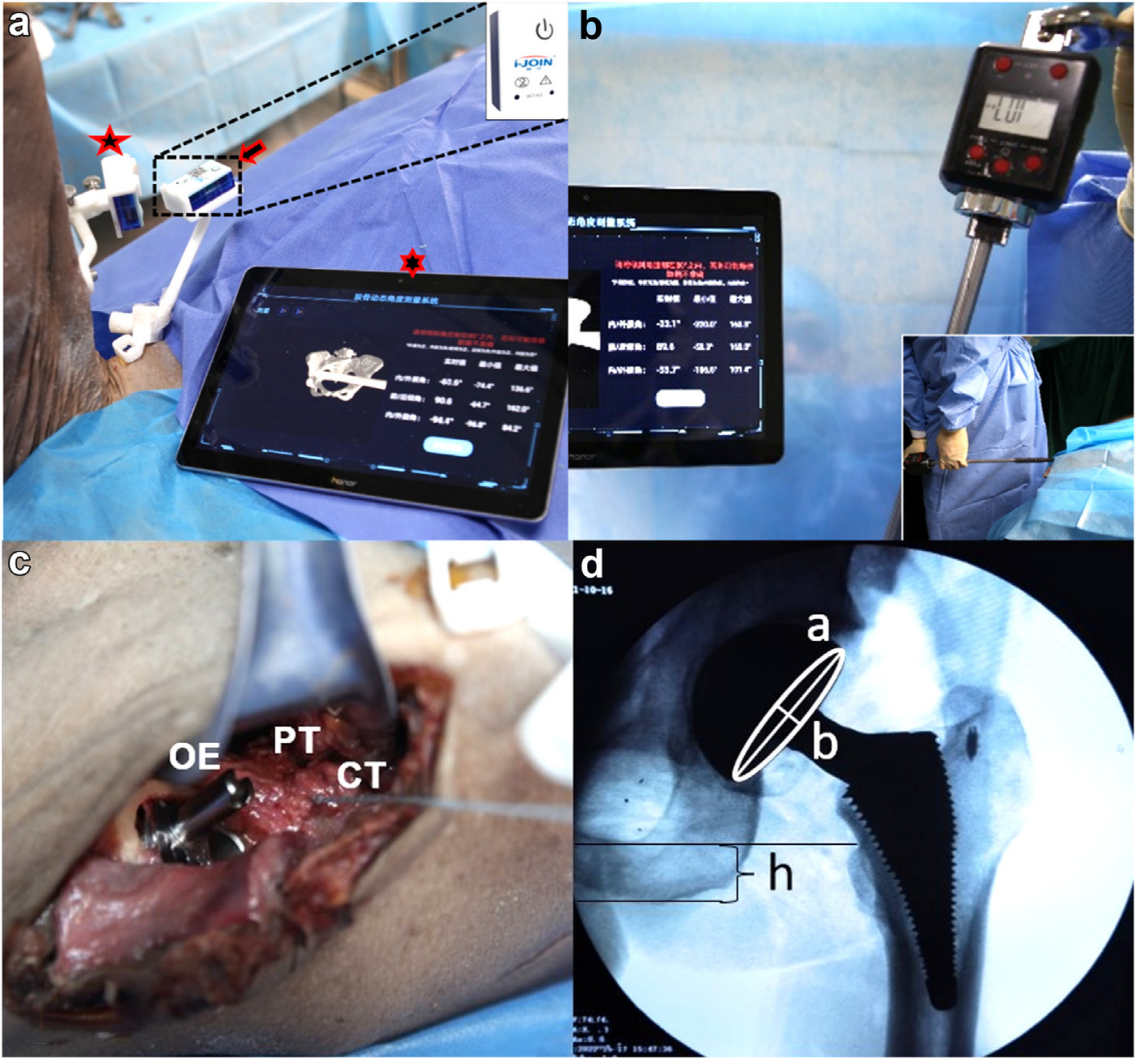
(a) I-joint Mini Navigation System. The pentagon is the measurement module, the arrow points to the reference module, and the hexagon is the monitor. (b) Torque testing for hip dislocation. (c) Anchor repair of the joint tendon. (d) Intraoperative X-ray fluoroscopic evaluation. a: Long axis of the acetabular projection; b: short axis of the acetabular projection; h: distance from the lesser trochanter to the sciatic bone.
